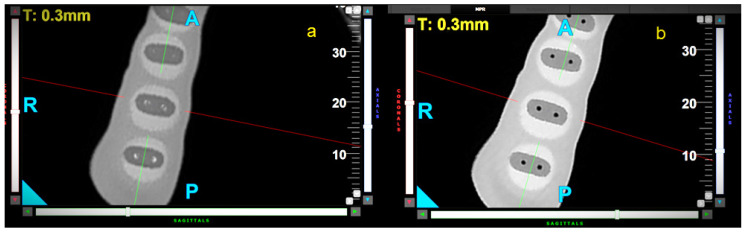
Representative CBCT axial—horizontal slices of samples at 9 mm from the apex, before (a) and after (b) canal preparation—OTR movement. The letters A, P, R indicate sides—anterior, posterior, and right respectively.
